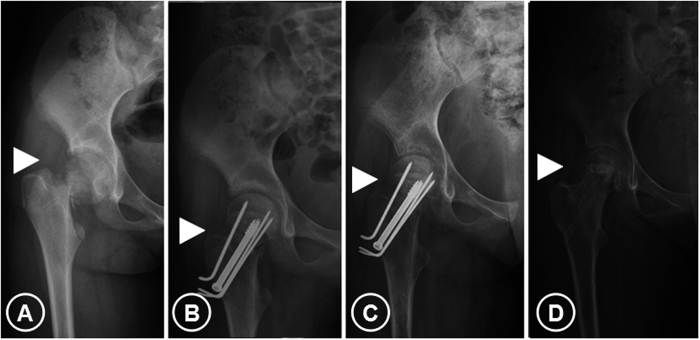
The first patient, a 13-year-old girl: one case of osteonecrosis after femoral neck fracture. (A) X-ray showing displaced femoral neck fracture (Delbet Type II). (B) X-ray image after internal fixation. (C) X-ray image 15 months after internal fixation showing good healing of femoral neck fracture segments after surgery; then she underwent the surgery for internal fixation removal. (D) X-ray image 2 months after internal fixation removal when she felt pain in her right hip showing the necrotic lesion of right femoral head and sclerosis zone formation around the necrotic bone (arrowheads).
